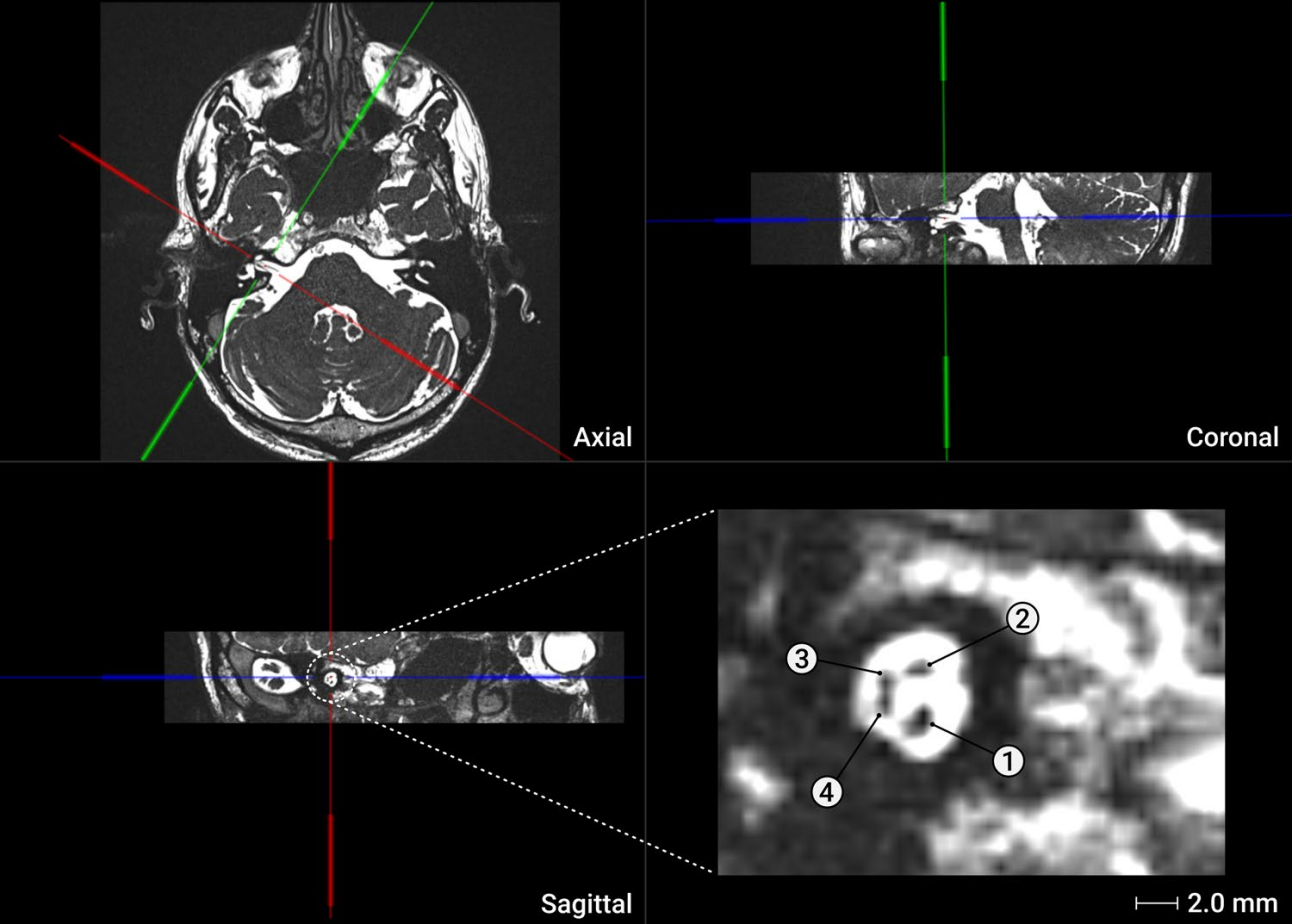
Cochlear and facial nerve measurement. A 3D CISS MRI sequence was employed to examine the petrous bone of a 45-year-old adult. The resulting image was aligned axially, coronally, and sagittally, revealing an enlarged right internal auditory canal housing the cochlear nerve (1), facial nerve (2), and superior (3) and inferior vestibular nerves (4). Software tools were utilized to measure the longest and widest segments of the cochlear and facial nerves. This figure description is adapted from OTOPLAN software (CAScination AG, Bern, Switzerland, version 3)
